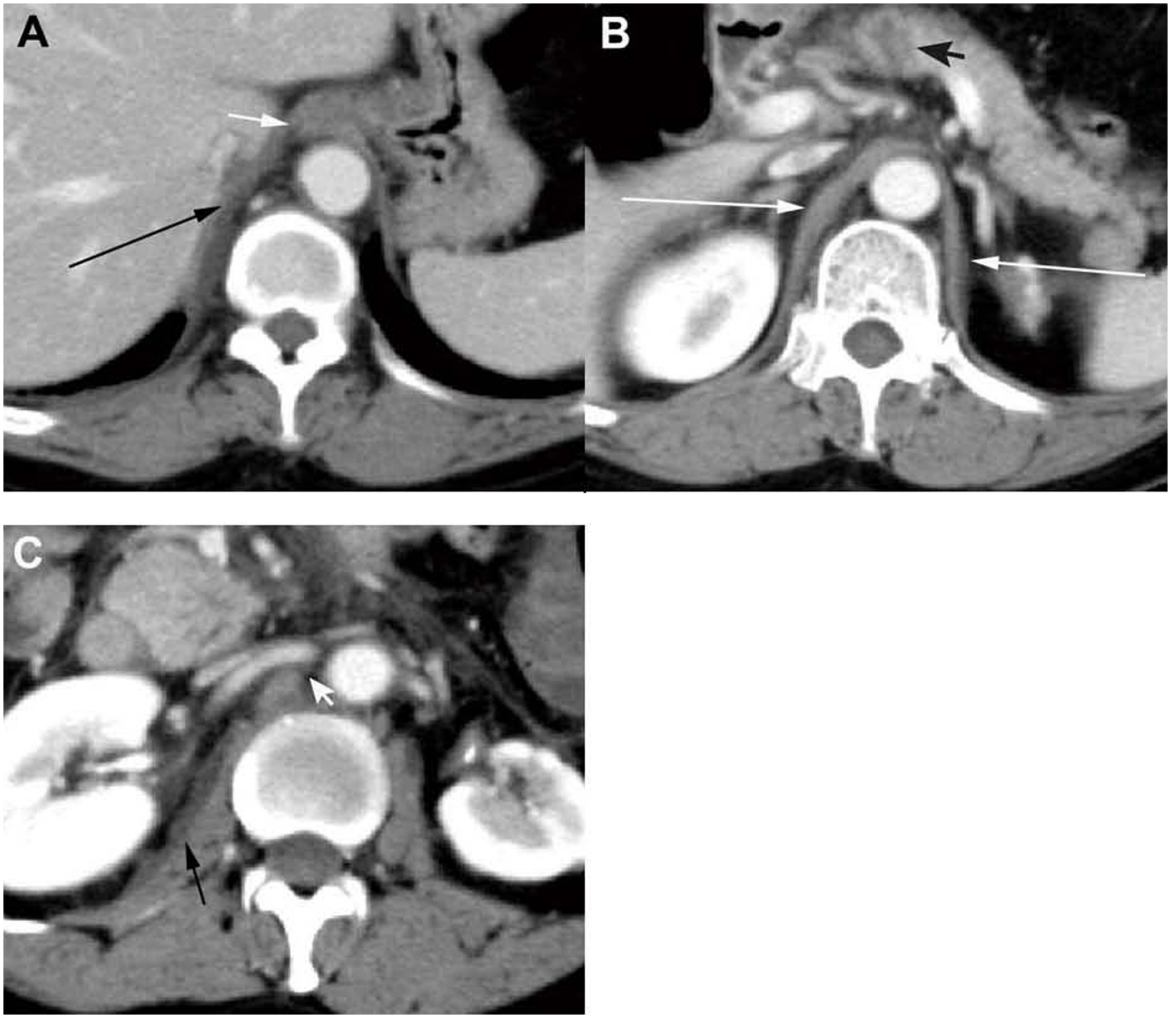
CT scans showing peripancreatic fluid draining into retrocrural space in a 46-year-old woman with AP. (A) On the upper section, CT scans obtained 4 days after admission showed the peripancreatic fluid had drained into the retrocrural space across the esophageal hiatus (*short white arrow*); then formed a fistulous tract into pleural cavity and developed into the pleural effusion (*long black arrow*). (B) On the middle section, pancreatic head necrosis is shown as a non-enhanced area that was less than 30% of total pancreatic area (*black arrowhead*). The bilateral diaphragmatic crus were shown hydropsia (*long white arrow*). (C) On the lower section, CT scans show the fluid had extended into the right retroperitoneal space (*short black arrow*) and further drained into the retrocrural space across the aortic hiatus (*white arrowhead*).
